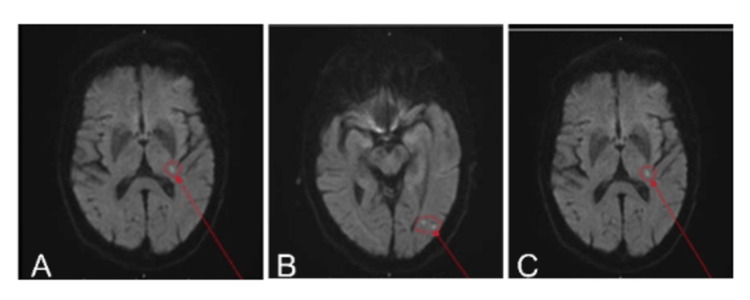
MRI brain images showing infarct regions. (A) Axial fluid-attenuated inversion recovery (FLAIR) highlighting periventricular lesion (circled and arrowed). (B) Axial FLAIR showing left occipitotemporal lesion (circled and arrowed). (C) Axial diffusion-weighted image demonstrating left thalamocapsular infarct (arrow). (D) Blank panel placeholder. The circle and arrow show the infarct.
